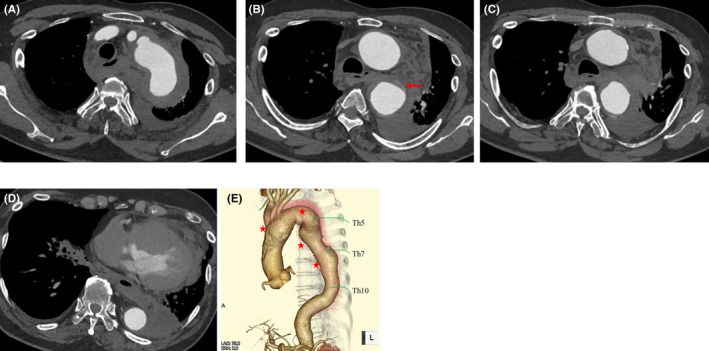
Preoperative computed tomography. Computed tomography shows type B acute intramural hematoma with rupture in the descending aorta. (A) 5th thoracic vertebra. Thrombosed false lumen of the aorta ranges from the arch to the descending aorta at the 7th thoracic vertebra. (B) 6th thoracic vertebra. The bleeding site seems to be located at the level of the 6th thoracic vertebra (arrow). (C) 7th thoracic vertebra. Hemothorax is observed in the left pleural cavity. The site of primary entry and ulcer‐like projections is unclear. (D) 10th thoracic vertebra. The descending aorta at the level of the 10th thoracic vertebra is normal in size and shape without dissection. (E) Three‐dimensional images reconstructed by computed tomography. Aortic aneurysms in the middle and distal aortic arch and descending aorta with diameters of 50 mm and 40 mm (four stars), respectively
